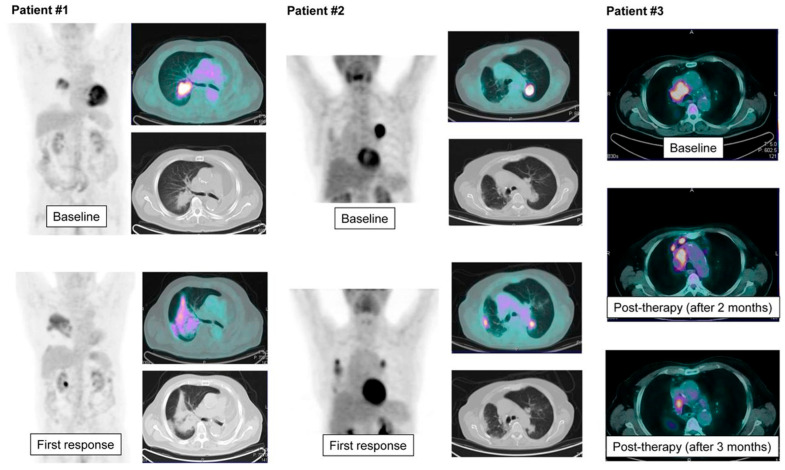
Representative FDG PET/CT images of 3 patients showing discordant response at CT-based and FDG PET/CT-based evaluation or between PERCIST and imPERCIST. Patient 1 was classified as PD with RECIST because of significant increase in dimensions of perihilar lesion in right lung; however, marked reduction in peak SUL allowed us to classify this patient as PMR according to both PERCIST and imPERCIST. In contrast, patient 2 showed marked lesion shrinkage, but lesion metabolism was substantially stable (possibly from inflammatory infiltration) or even mildly increased in the case of right pleural metastasis. This patient was classified as SMD with both PERCIST and imPERCIST, but the response was more evident on CT images. Patient 3 was differently classified by PERCIST and imPERCIST. At first response (2 mo after therapy), he was classified as SMD by imPERCIST; however, because of new lesions, was considered PERCIST PMD. After 1 mo more, new lesions disappeared and lesions present since baseline showed a significant reduction in both dimensions and metabolism. (Reprinted/adapted with permission from Ref. [71]. Copyright © Society of Nuclear Medicine and Molecular Imaging).
